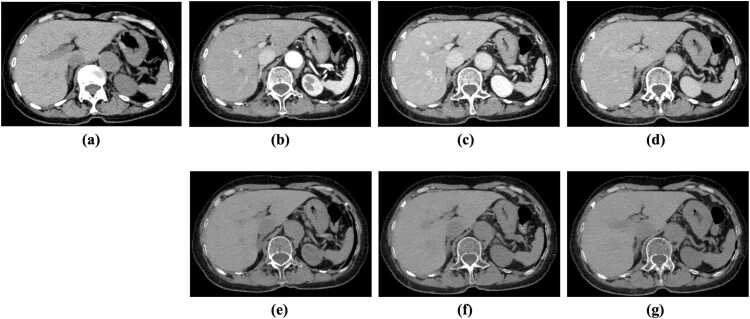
One of the clinical cases with TNC image and VNC images for quantitative evaluation created from the three contrast-enhanced phases. A woman who was 72 years when image. (a) TNC, (b) AP, (c) PVP, (d) EP, (e) VNC(AP), (f) VNC(PVP), (g) VNC(EP). The CT value of the liver parenchyma on the TNC image was 70.7. The CT values on the VNC images were 65.3 on VNC(AP), 63.7 on VNC(PVP), and 64.3 on VNC(EP).
